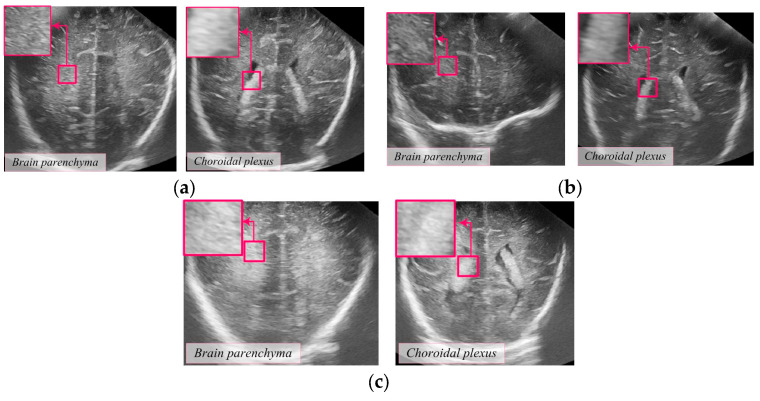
Ultrasound images of brain parenchyma and choroid plexus (magnification: 3×): (a) Normal, (b) Moderate, and (c) Intensive.
